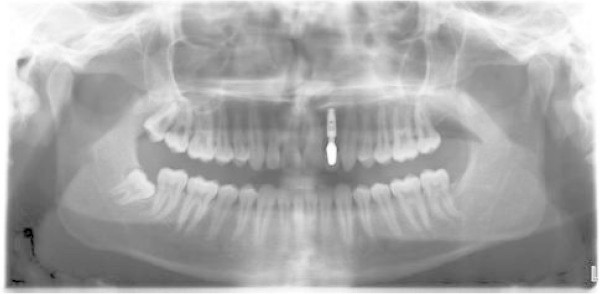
Post-treatment panoramic X-ray.
